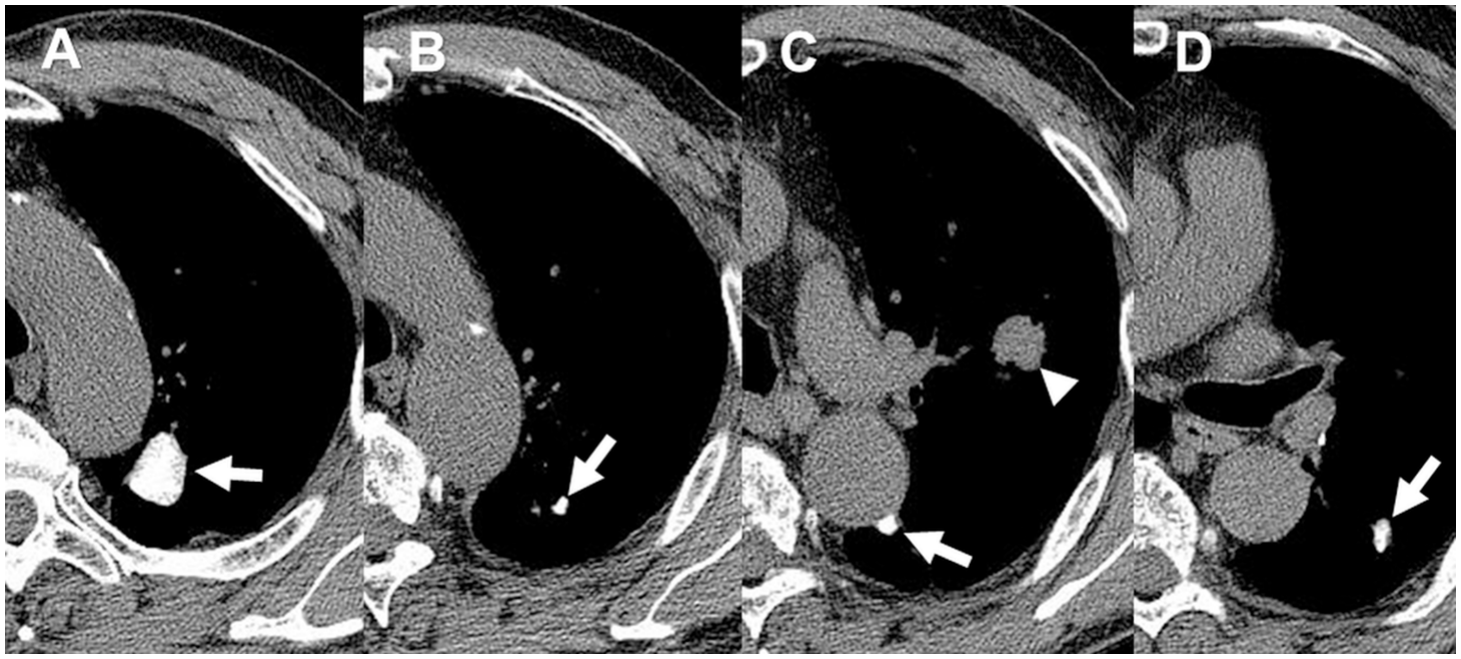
A 78-year-old men with squamous cell carcinoma in the left upper lobe. (A-D) Non-contrast-enhanced chest CT images demonstrate multiple calcified nodules in the left lung (arrows). (C) CT image demonstrates a nodule (arrowhead) in the left upper lobe. He underwent left upper lobectomy and systemic nodal dissection via thoracotomy. During the operation, there was severe pleural adhesion requiring seven hours for adhesiolysis.
